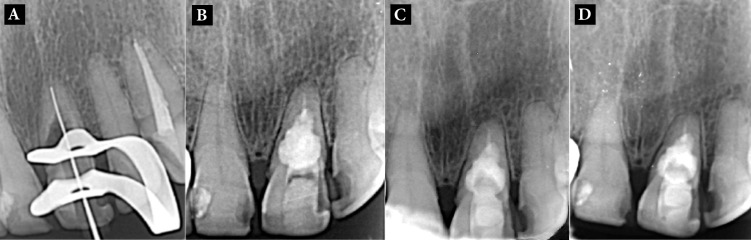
A)* Endodontic treatment and working length determination; *B)* Post-operative radiography, the root canal is filled with CEM cement; *C)* Six-month recall; *D)* One-year recall; the tooth is absolutely functional and symptom-free
